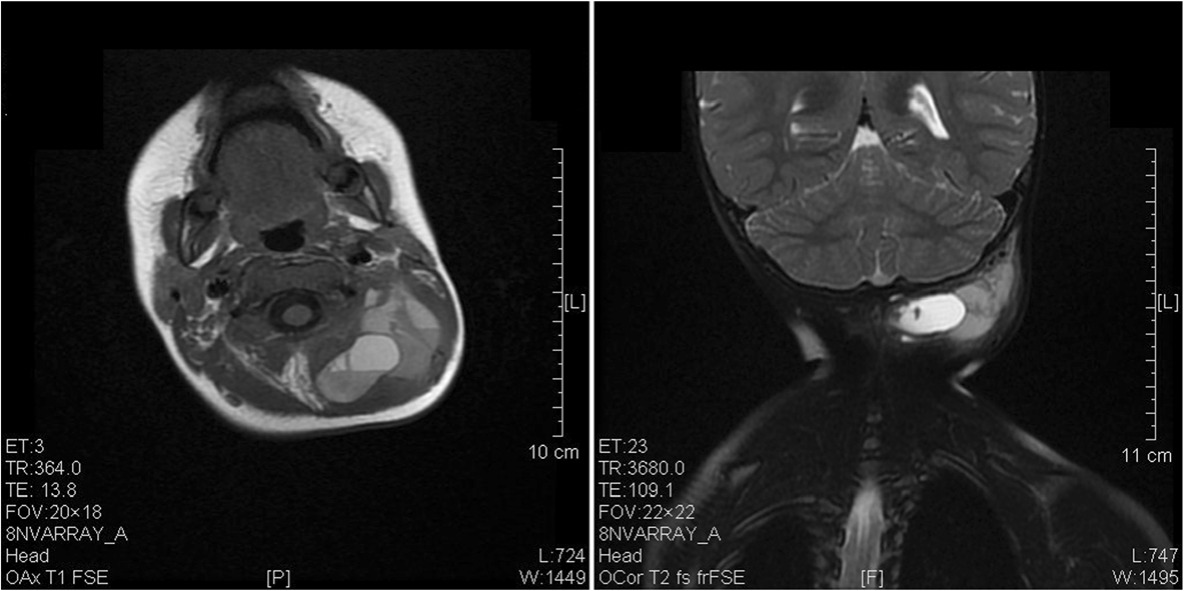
A magnetic resonance image showed an enlarged heterogenous mass in the left occipitalia in case 1.
